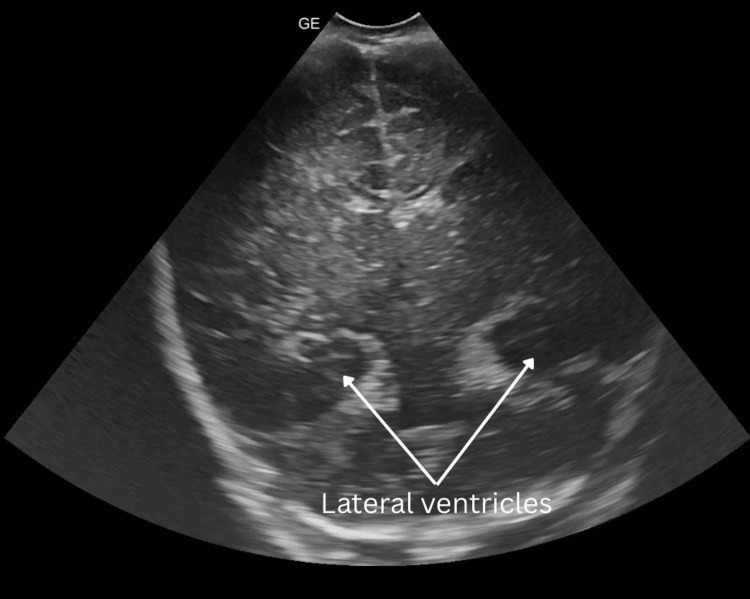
Brain ultrasound Brain ultrasound showed no obvious sonographic abnormality, with no evidence of intraventricular hemorrhage or ventriculomegaly.
